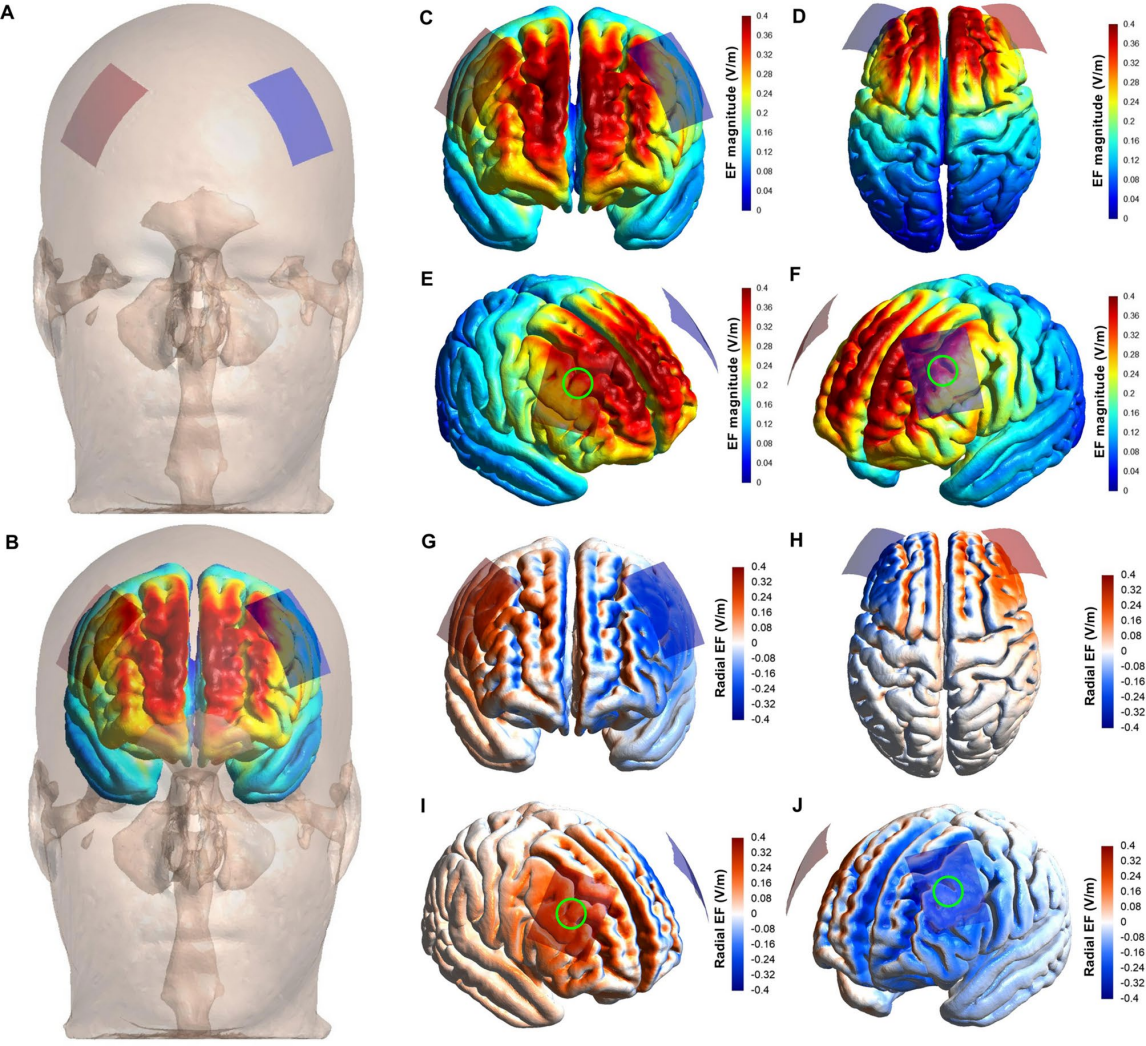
The electric field magnitude and radial component induced by the tDCS montage. Analysis of tDCS-induced strength and radial (normal to the cortical surface) component of the electric field (EF) using a head model (MNI152) developed from magnetic resonance imaging. Electrode montage targeting anodal tDCS in the right dorsolateral prefrontal cortex. Anodal (red rectangle; 5 × 4 cm) and cathodal (blue rectangle; 5 × 4 cm) electrodes were placed over the scalp (A and B). The magnitude of the EF is shown in panels C-F, with hot colors (e.g., red) representing stronger EF and cold colors (e.g., blue) representing weaker EF. Panels G-J depict the radial EF, with red representing the electric current flowing into the cortex (i.e., inducing excitatory effects) and blue representing the electric current flowing out of the cortex (i.e., inducing inhibitory effects). Panels E and F show that the research montage reached the target area with enough electric current magnitude to generate a neuromodulatory effect (green circles roughly indicating the target locations). Furthermore, as shown in panels I and J, the target areas were stimulated with the desired polarity (i.e., anodal current) to elicit excitatory effects in the target regions (green circles roughly indicating the target areas)
